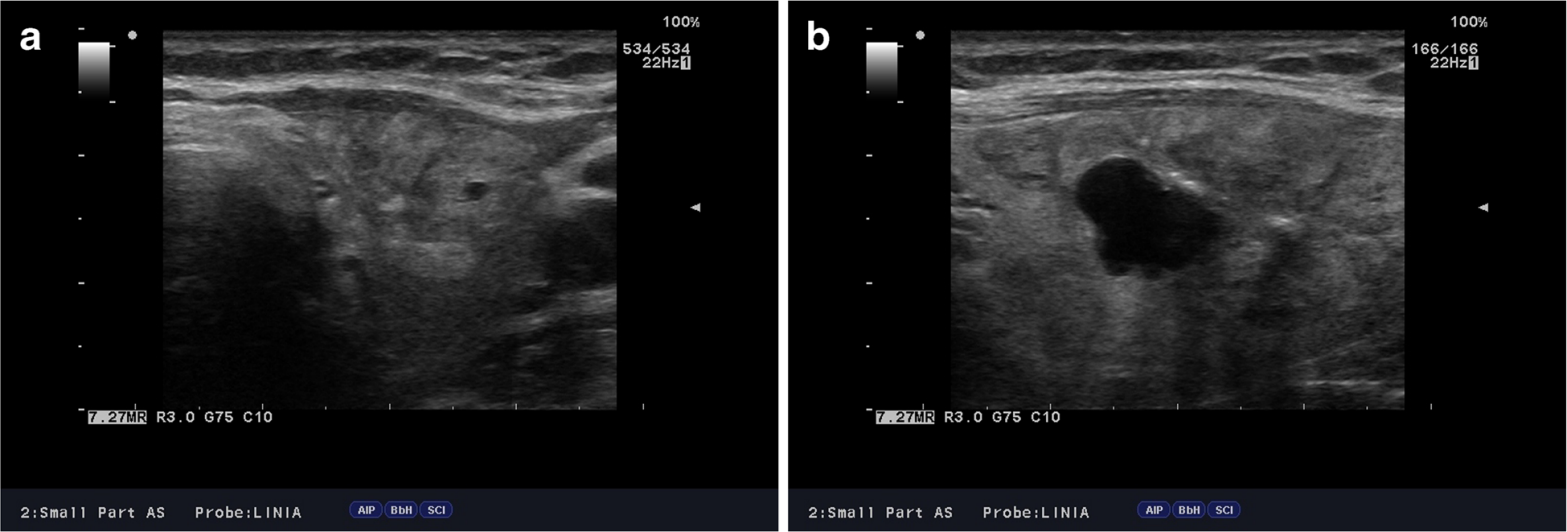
(a,b) Ultrasound image of left thyroid lobe with the infiltration of small lymphocytic B-cell lymphoma in 57-year-old female with SLL-B/CLL-B.
